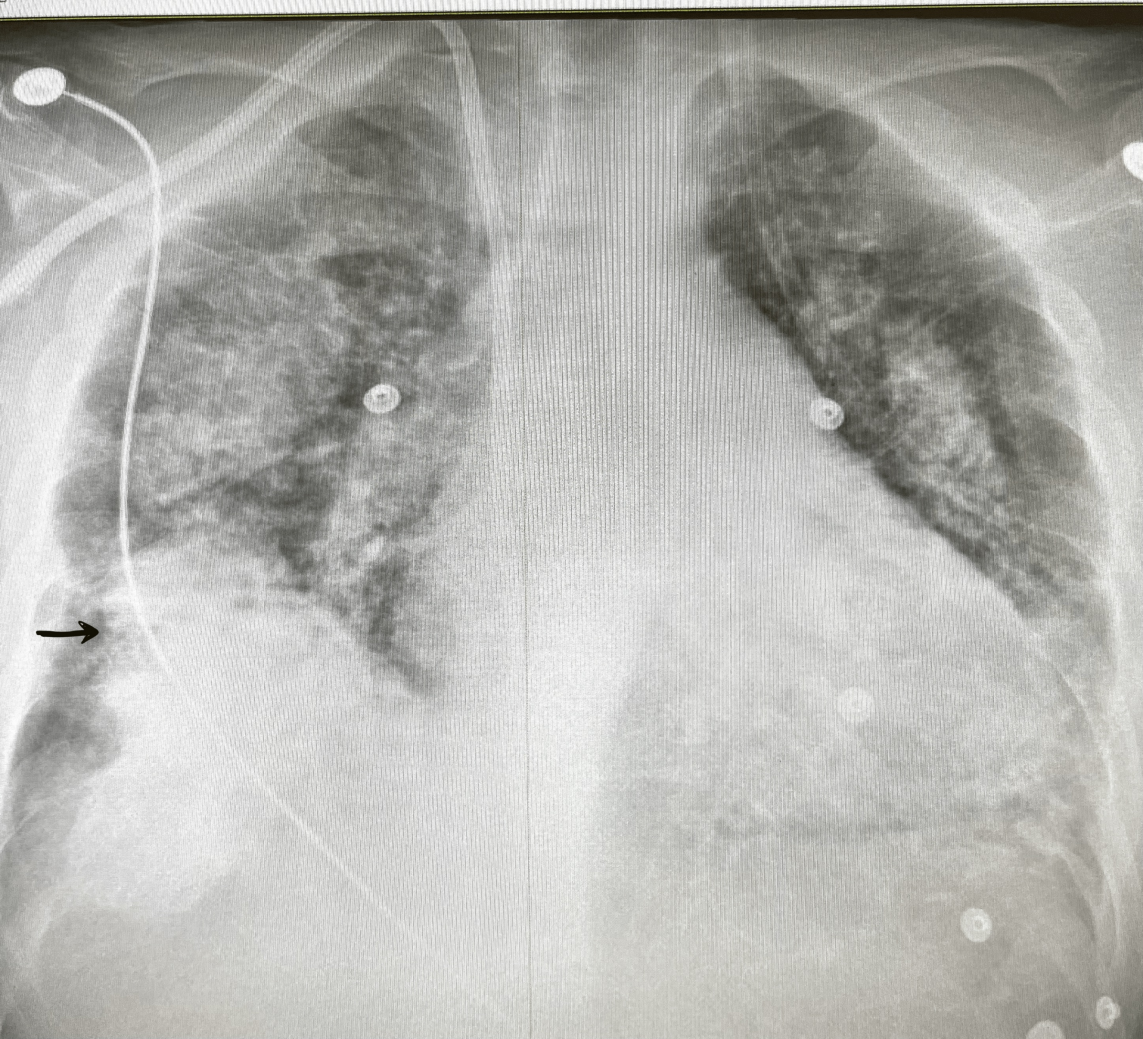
Chest radiograph showing bilateral patchy opacities This finding is non-specific and was initially treated as a possible infection, but later correlated with CT and bone scintigraphy, supporting a diagnosis of metastatic pulmonary calcification.
